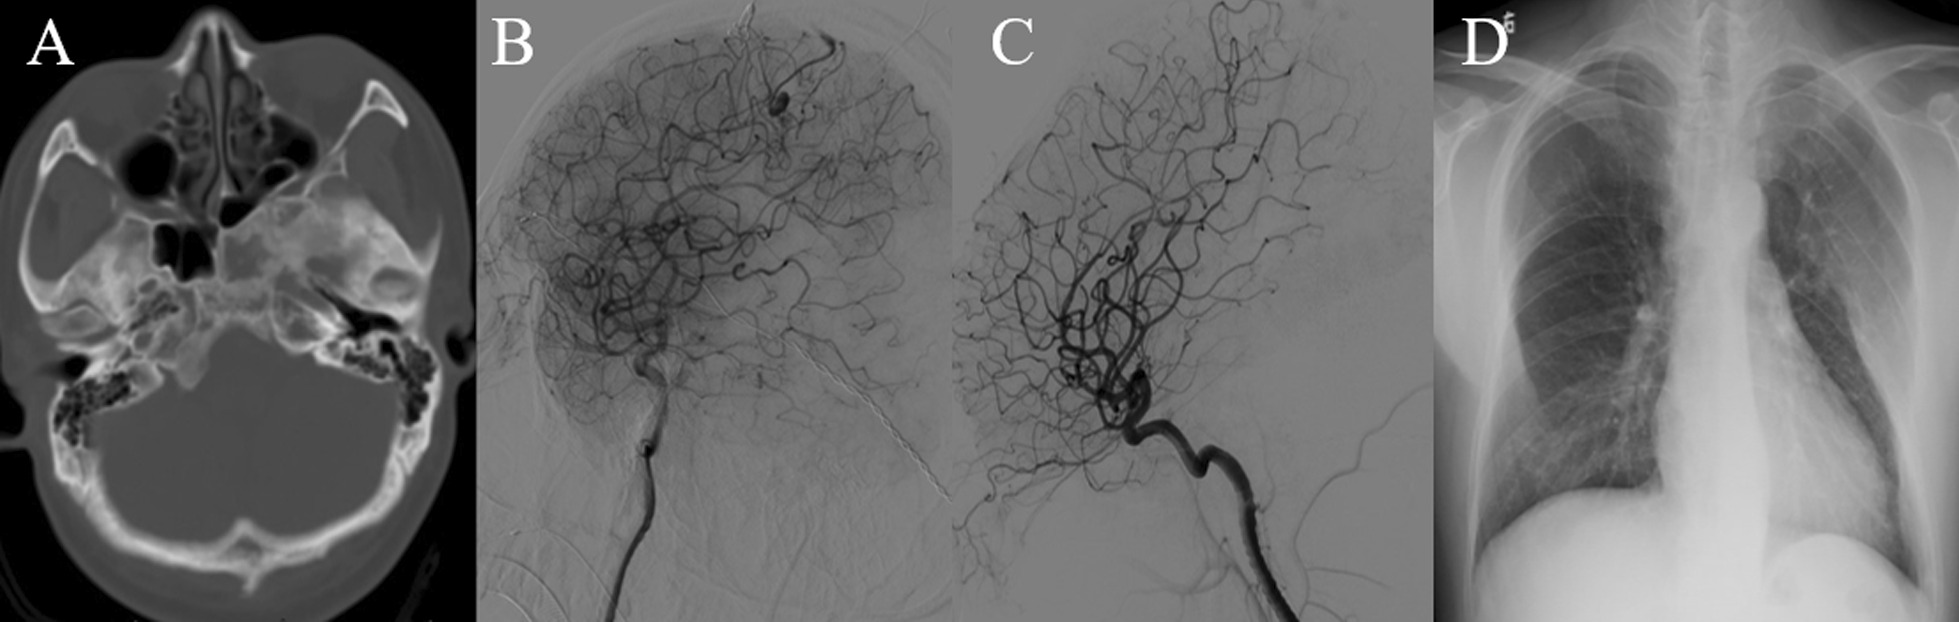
CT showing FD of the left sphenoid bone (A). DSA showing arteriovenous malformation in the right parietal lobe with right anterior cerebral artery being the major feeding artery and superior sagital sinus drainging (B). The lesion was resected completely (C). Chest X-ray showing FD of multiple ribs (D)
